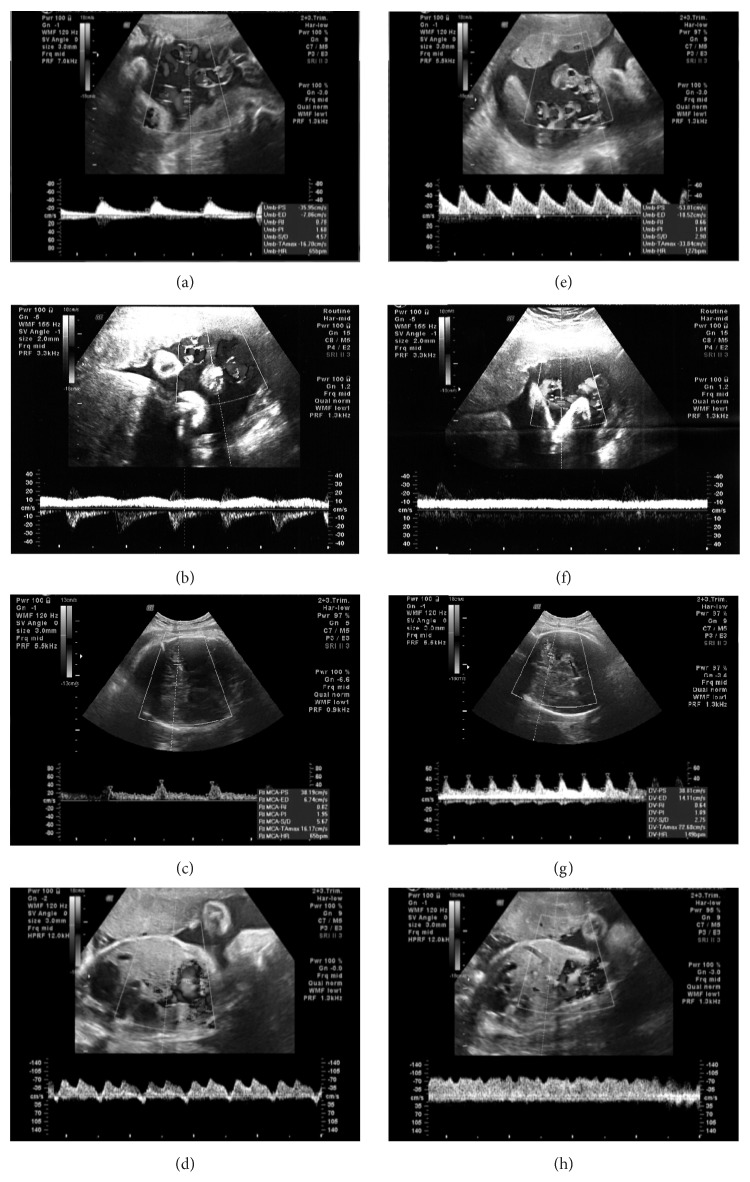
Umbilical artery (a), umbilical vein (b), middle cerebral artery (MCA) (c), and ductus venosus (d) Doppler velocimetry during the deceleration period and Doppler velocimetry of these vessels at normal heart rate, accordingly (e, f, g, h).
